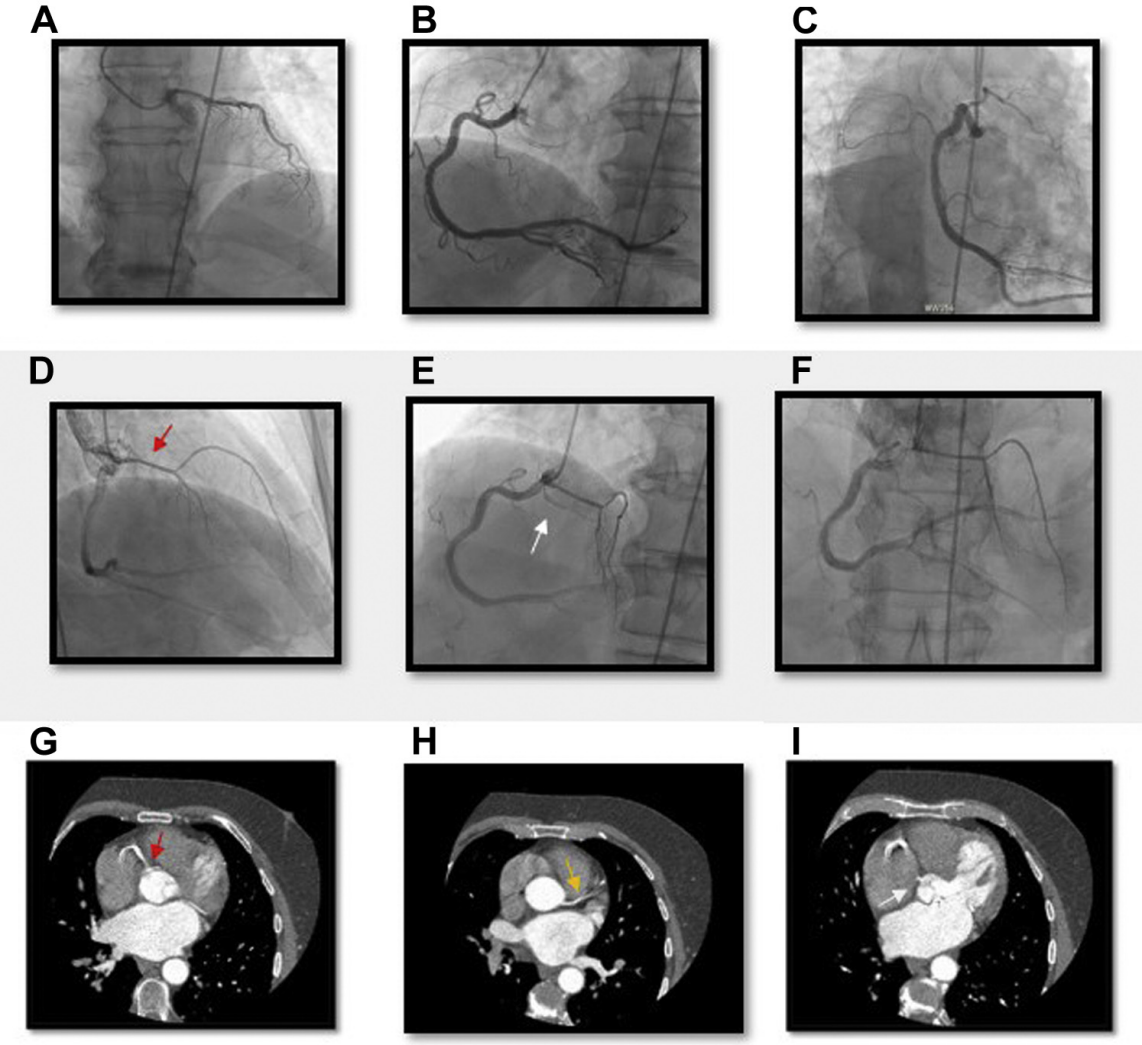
Coronary angiography and computed tomography angiography demonstrating multiple coronary anomalies. (A) Secondary LAD artery from the left coronary cusp using the JL3.5 in the RAO view. (B) RCA selectively engaged in the LAO view. (C) RCA selectively engaged in the RAO caudal view. (D) RCA with the principal LAD artery (red arrow) in the RAO cradial view. (E) RCA angiogram in the LAO cranial view revealing all 3 right-sided vessels including the LCx artery (white arrow). (F) RCA angiogram revealing the principal LAD artery branching from the RCA in the AP cranial view. (G) Principal LAD artery (red arrow) branching from the RCA. (H) Secondary LAD artery (yellow arrow) branching from the left coronary cusp. (I) LCx artery with a separate ostium branching from the RCC. AP, anteroposterior; LAD, left anterior descending; LAO, left anterior oblique; LCx, left circumflex; RAO, right anterior oblique; RCA, right coronary artery; RCC, right coronary cusp.
